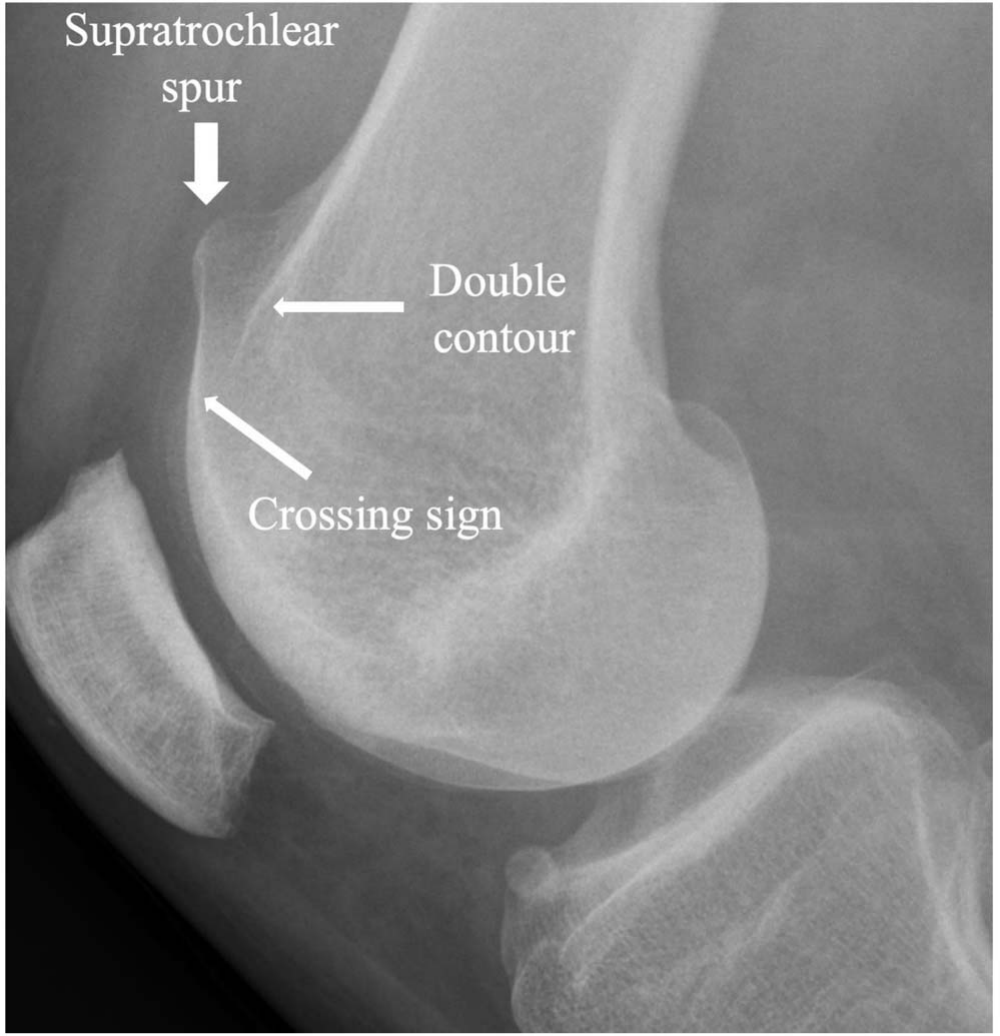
Three pillars of trochlear dysplasia visible on X-rays.
